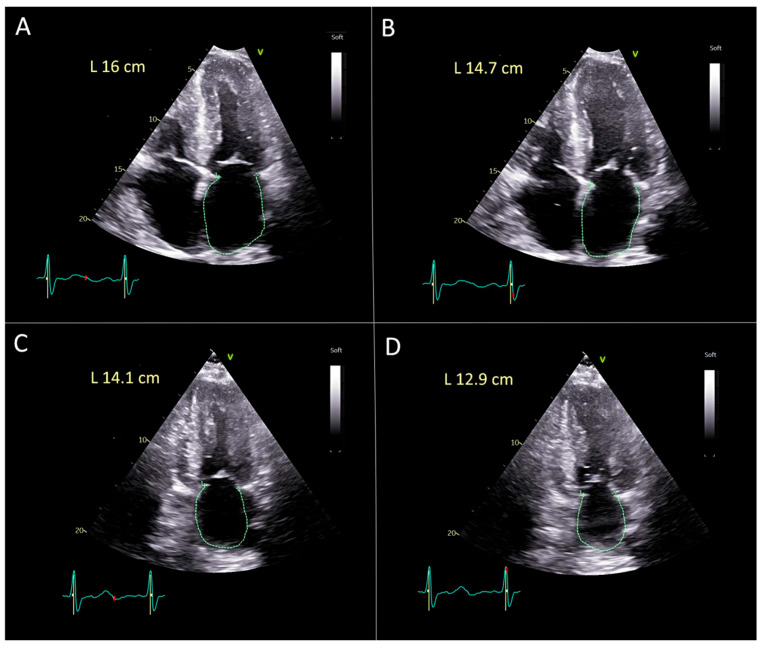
Manual measurements of biplane average peak reservoir left atrial strain. (A) Apical 4-chamber view during systole: maximal left atrial length (Ls4c) = 16.0 cm. (B) Apical 4-chamber view during diastole: minimal left atrial length (Ld4c) =14.7 cm. (C) Apical 2-chamber view during systole: maximal left atrial length (Ls2c) =14.1 cm. (D) Apical 2-chamber view during diastole: minimal left atrial length (Ld2c) = 12.9 cm. Manual biplane left atrial reservoir strain (LASr) is calculated using the following formula: Biplane LASr (%) = [((Ls4c − Ld4c)/Ls4c) + ((Ls2c − Ld2c)/Ls2c)]2 × 100. Substituting the values = [((16.0 − 14.7)/16.0) + ((14.1 − 12.9)/14.1)]2 × 100 = [(1.3/16.0) + (1.2/14.1)]2 × 100 ≈ (0.08125 + 0.08511)/2 × 100 ≈ 0.08318 × 100 ≈ 8.3%. This value represents the manually measured biplane LASr.
